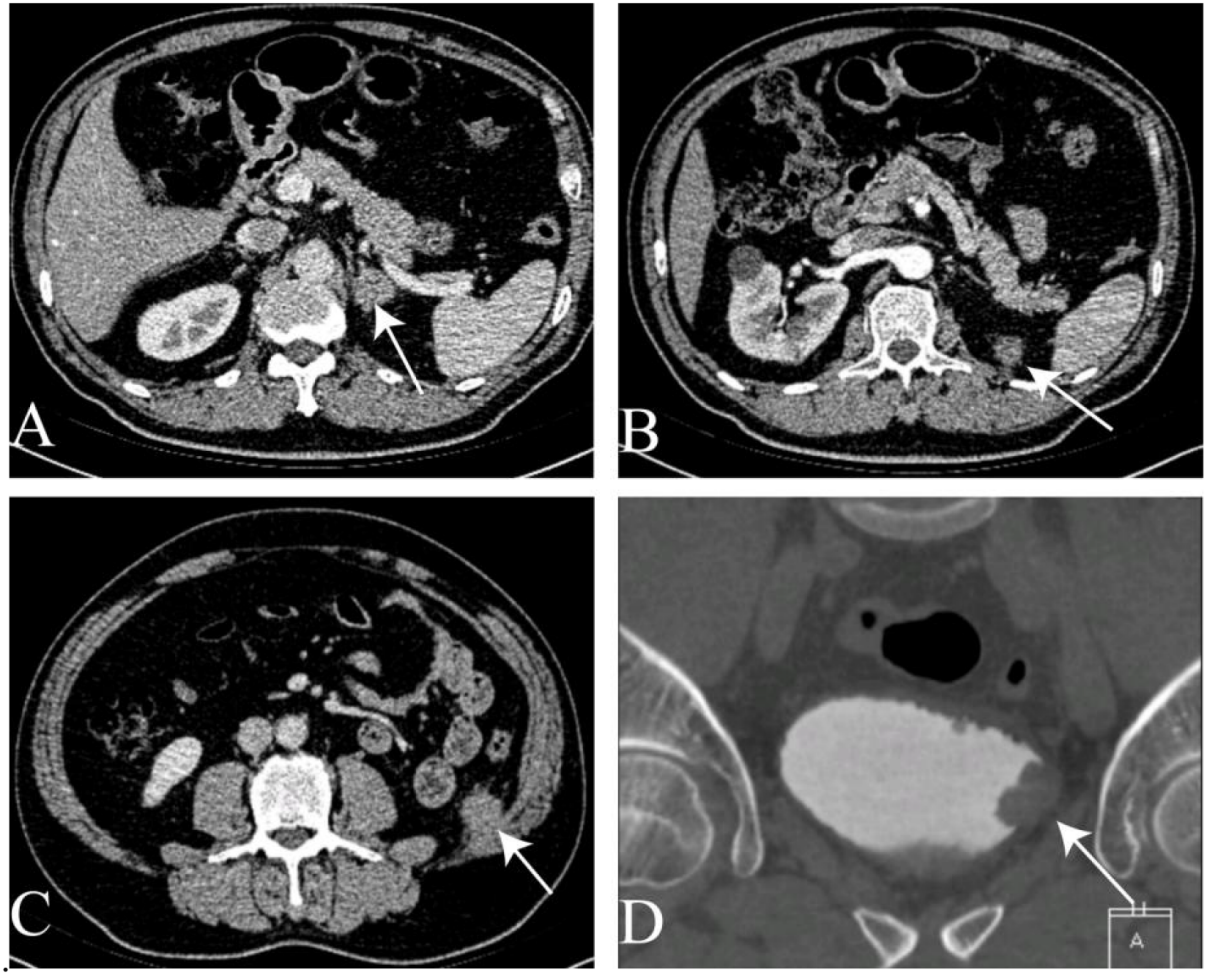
Computed tomography of the abdomen. (A) Left adrenal mass. (B) Mass in the left retroperitoneum and peritoneum. (C) Mass in the abdominal wall. (D) Mass in the bladder.
